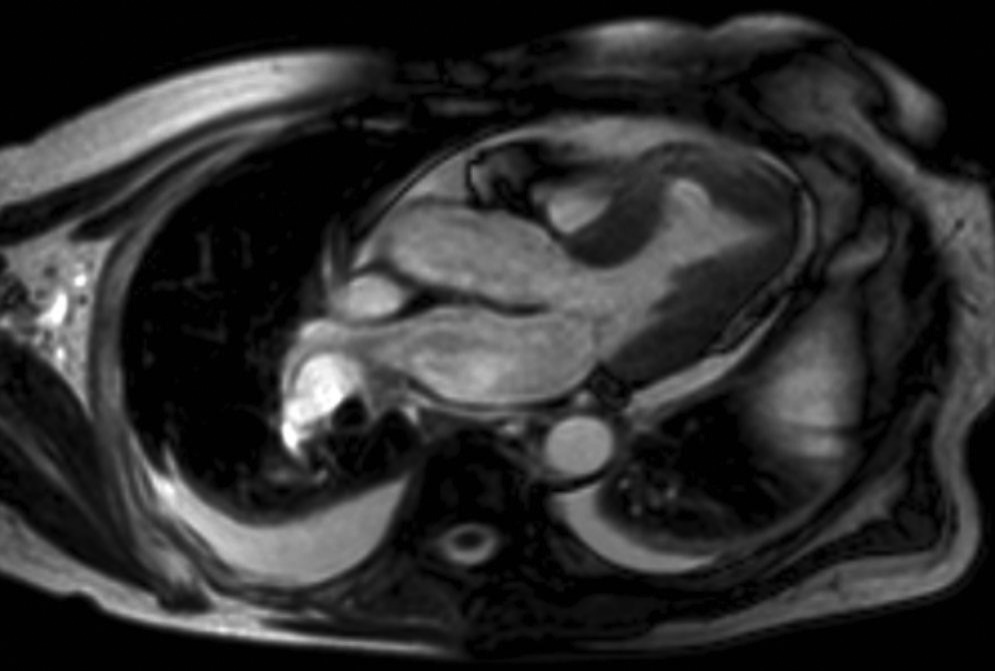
The aneurism containing the rupture can be seen on the distal septum (MRI image).
